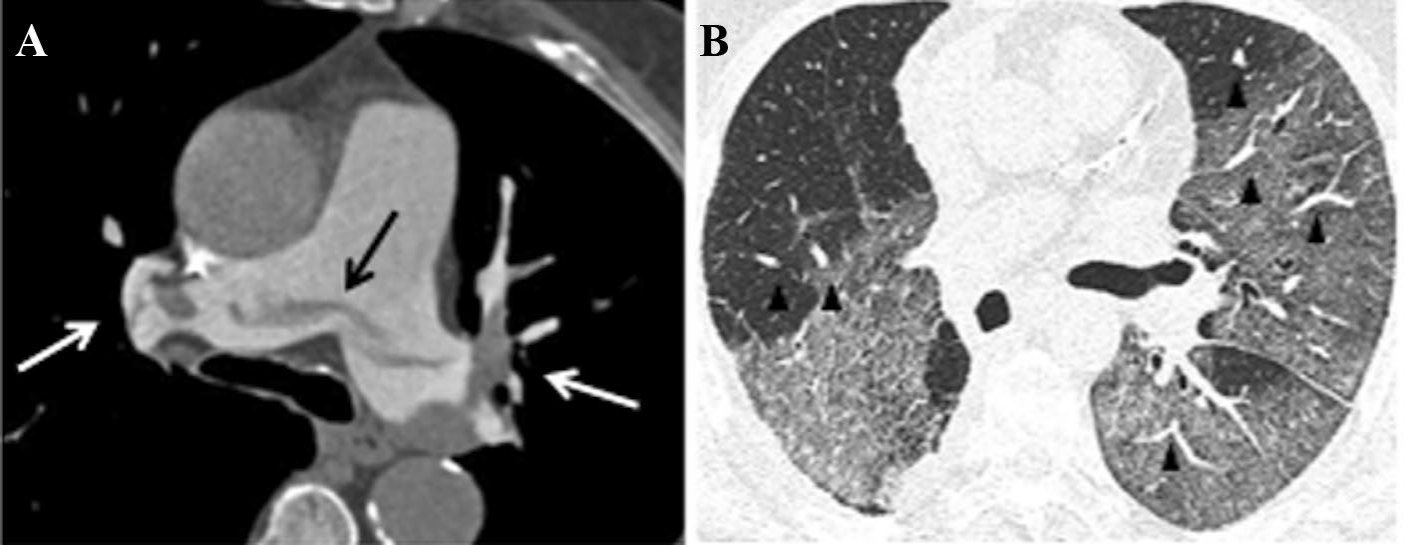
Pulmonary CT angiography of a 67-year-old male with acute pulmonary embolism. The CT scan was obtained six days after the onset of COVID-19 symptoms; on the same day the patient was transferred to the intensive care unit. A, Axial scan in mediastinal window shows linear saddle embolism of the pulmonary trunk (black arrow) and multiple bilateral filling defects involving lobar arterial branches (white arrows). B, Axial scans in lung window shows vascular enlargement (black arrowheads) and peripheral ground-glass opacities involving both lungs
